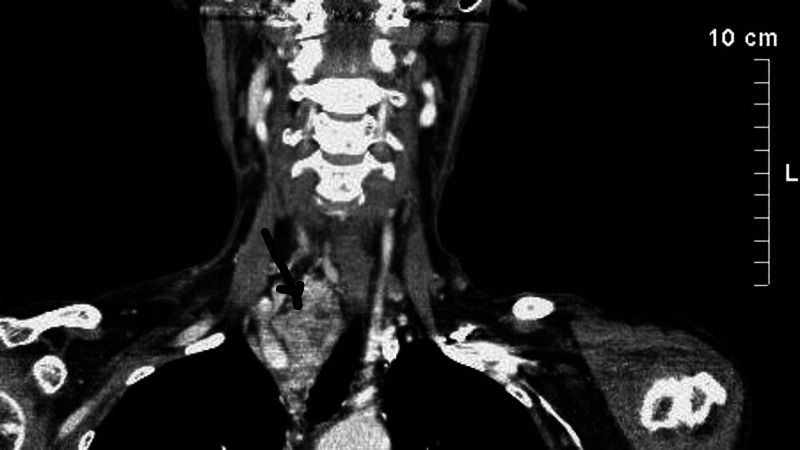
Three-dimensional CT angiography revealing blood supply of the mass The arrow indicates the position of the mass in the right supraclavicular fossa CT: computed tomography
